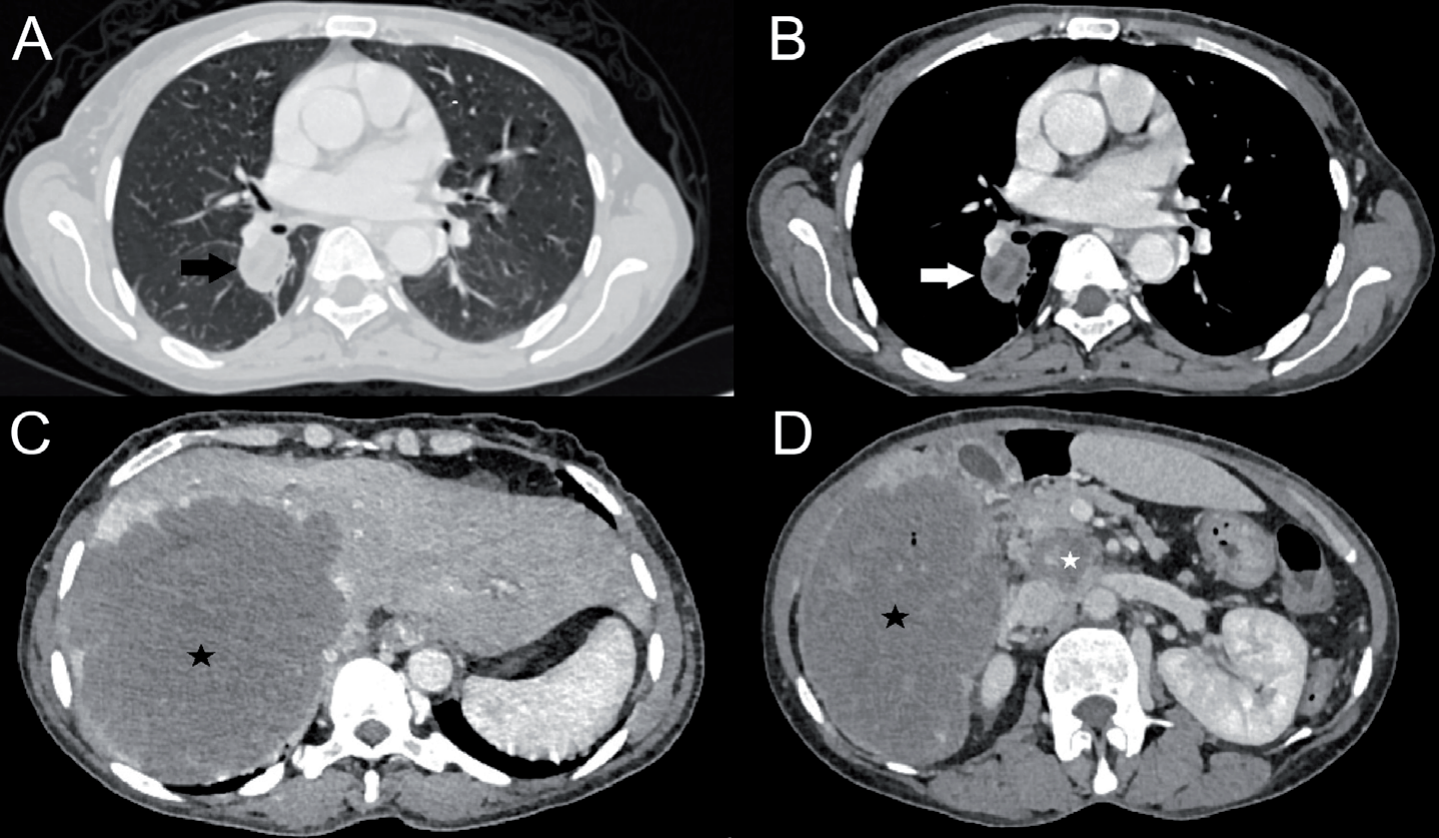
Axial contrast-enhanced computed tomography (CT) images at the level of the lung (A and B) and liver (C and D) show a large alveolar echinococcosis lesion located in the right lobe of the liver (black stars in C and D). Alveolar echinococcosis metastases in the right lung are shown in the same patient (black and white arrows in A and B). In addition, alveolar echinococcosis involvement is observed in the uncinate process of the pancreas (white star in D).
